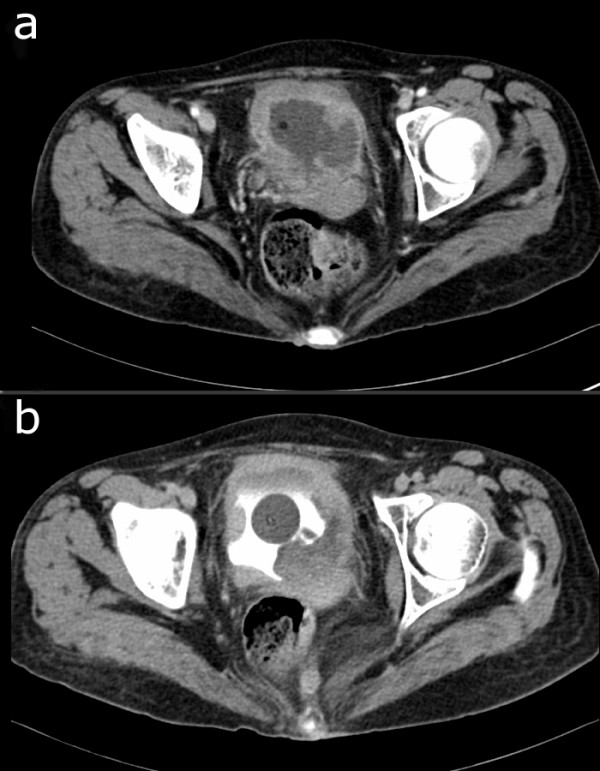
Computed tomography. Unenhanced (a) and enhanced (b) CT of pelvis showing diffuse extensive thickening of bladder wall.
